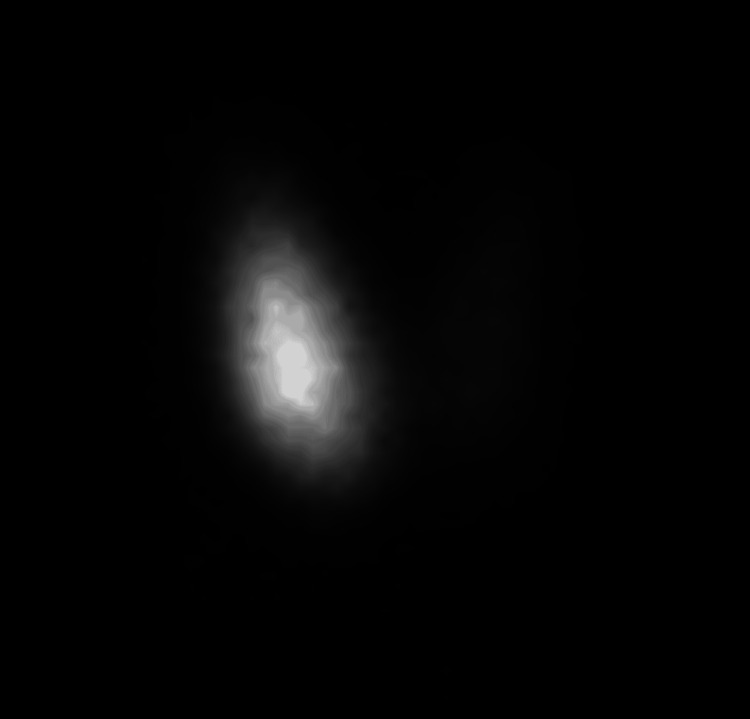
A 20-minute delayed anterior planar image of technetium-99m pertechnetate thyroid scintigraphy demonstrates an asymmetrically enlarged right thyroid lobe with relatively homogeneous dense radiotracer uptake and smooth outline. The left thyroid lobe is normal in size with homogeneous near-total suppression of radiotracer uptake.
